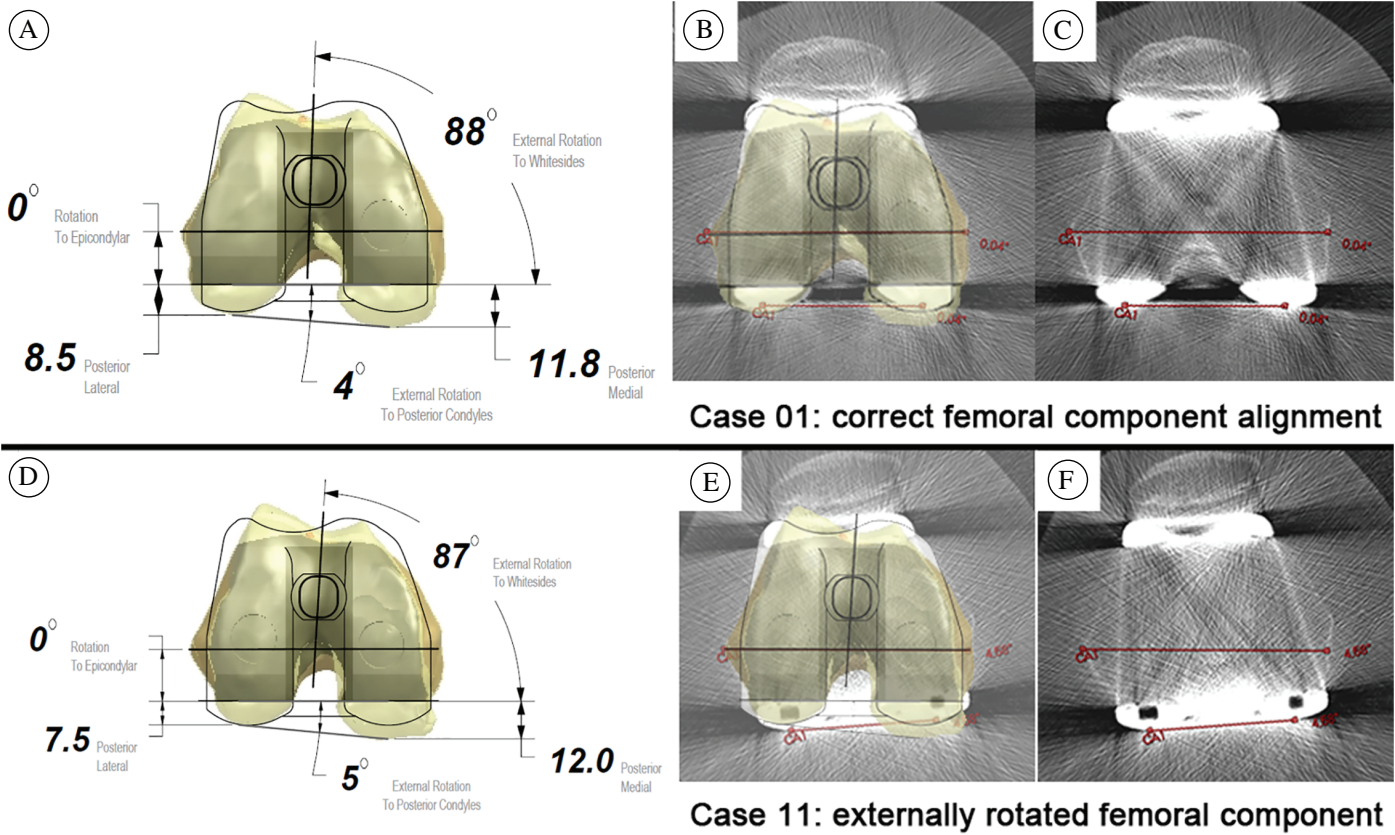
Preoperative planning (A, D), postoperative CT scans (C, F) and corresponding superimposition images (B, E) of femoral components in two cases: Case 01 (A–C): Correct alignment. Case 11 (D–F): Externally rotated component (4.48° mismatch from planning).
